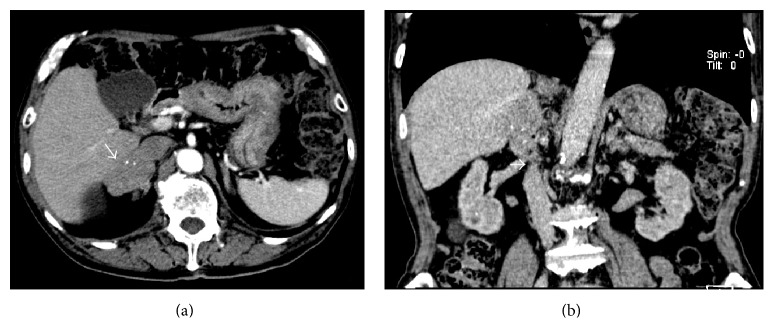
(a) Computed tomography revealed an enhanced lobular tumor (white arrow) with calcification in the region of the right adrenal gland; (b) the border of the tumor at the liver and the right adrenal gland was not clear, and the tumor was close to the inferior vena cava (IVC) (white arrow).
